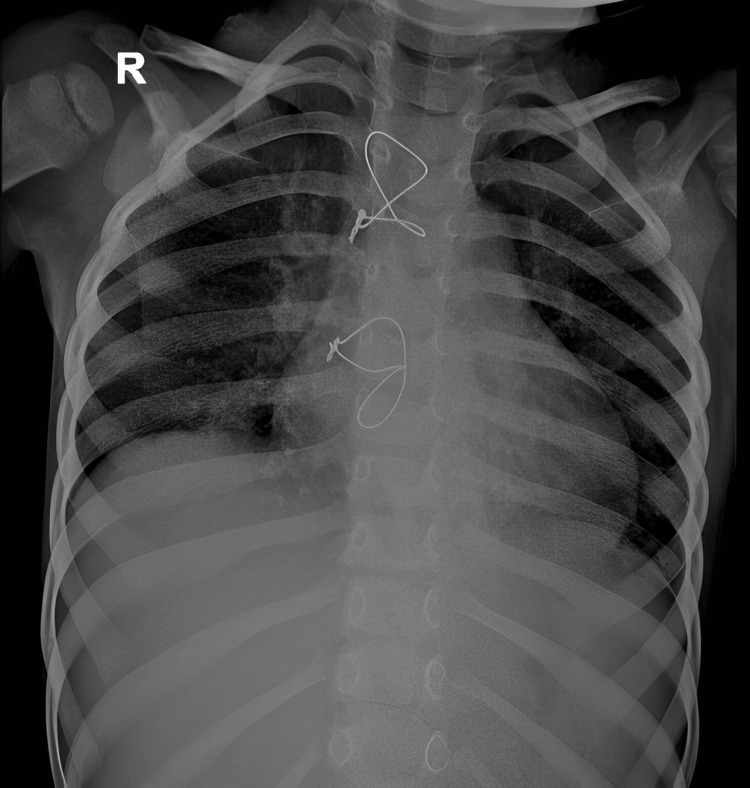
Chest X-ray demonstrating post sternotomy and bilateral hilar opacities with mild cardiomegaly
